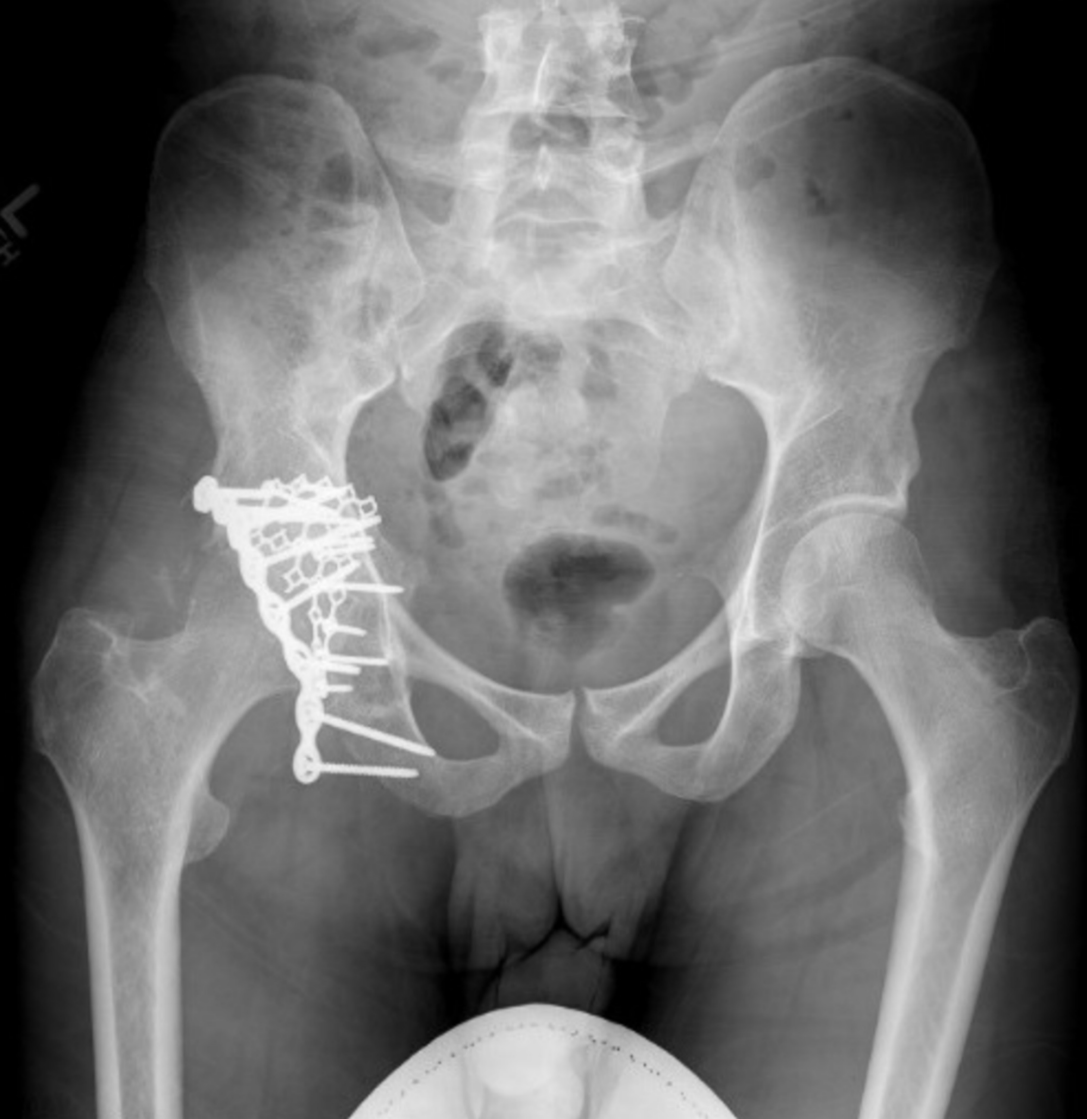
Anterior posterior X-ray of the pelvis demonstrating fixation of the right acetabulum following open reduction internal fixation and bone biopsy
